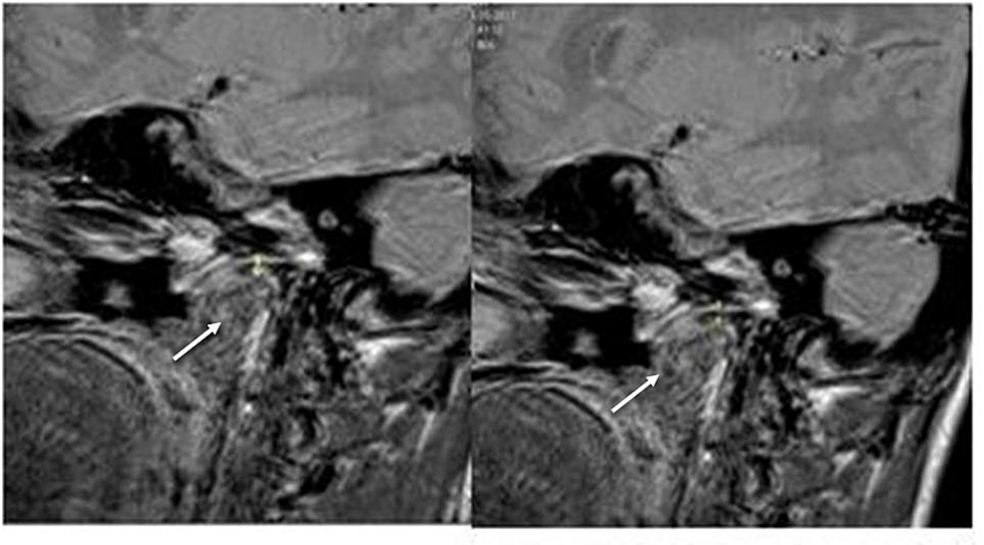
MRI images taken at T0 (left) and T1 (right) to demonstrate the dimensional changes of the condylar cartilage after twin-block therapy
